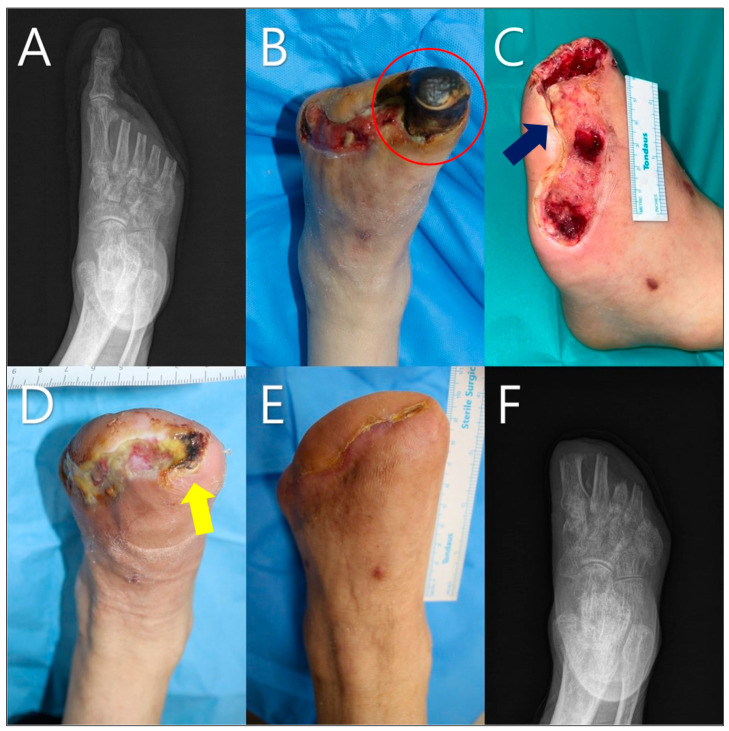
A 71-year-old male patient with a history of diabetes and angina. (A) Initial X-ray showing the extent of necrosis. (B) Pre-debridement image showing the necrotic great toe (red circle). (C) Post-debridement image showing exposed bone (blue arrow) before micronized acellular dermal matrices (mADMs) application. (D) Wound after initial mADM application, showing healing progress but with necrotic changes (yellow arrow). (E) Wound showing significant healing after a total of eight mADM applications, revision, and local flap. (F) Final X-ray showing no signs of bony and soft tissue complications, such as osteomyelitis, infection, or chronic inflammation.
